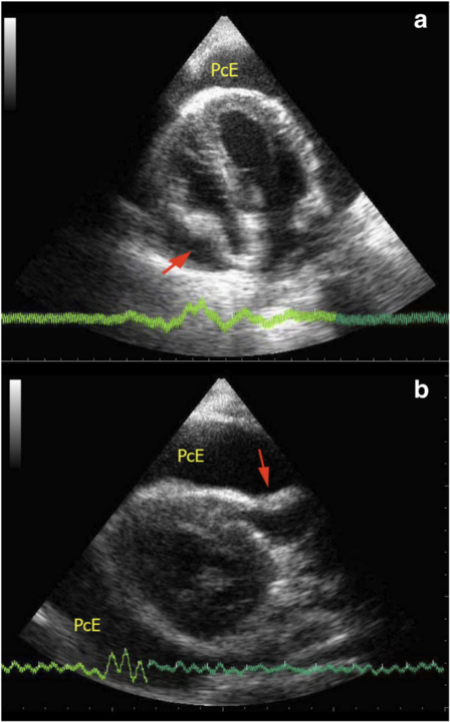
a, b Cardiac tamponade. These two images show collapsed RA and RV (*red arrows*) on apical four-chamber view and parasternal long axis view in a patient with cardiac tamponade (*PcE* = pericardial effusion)
